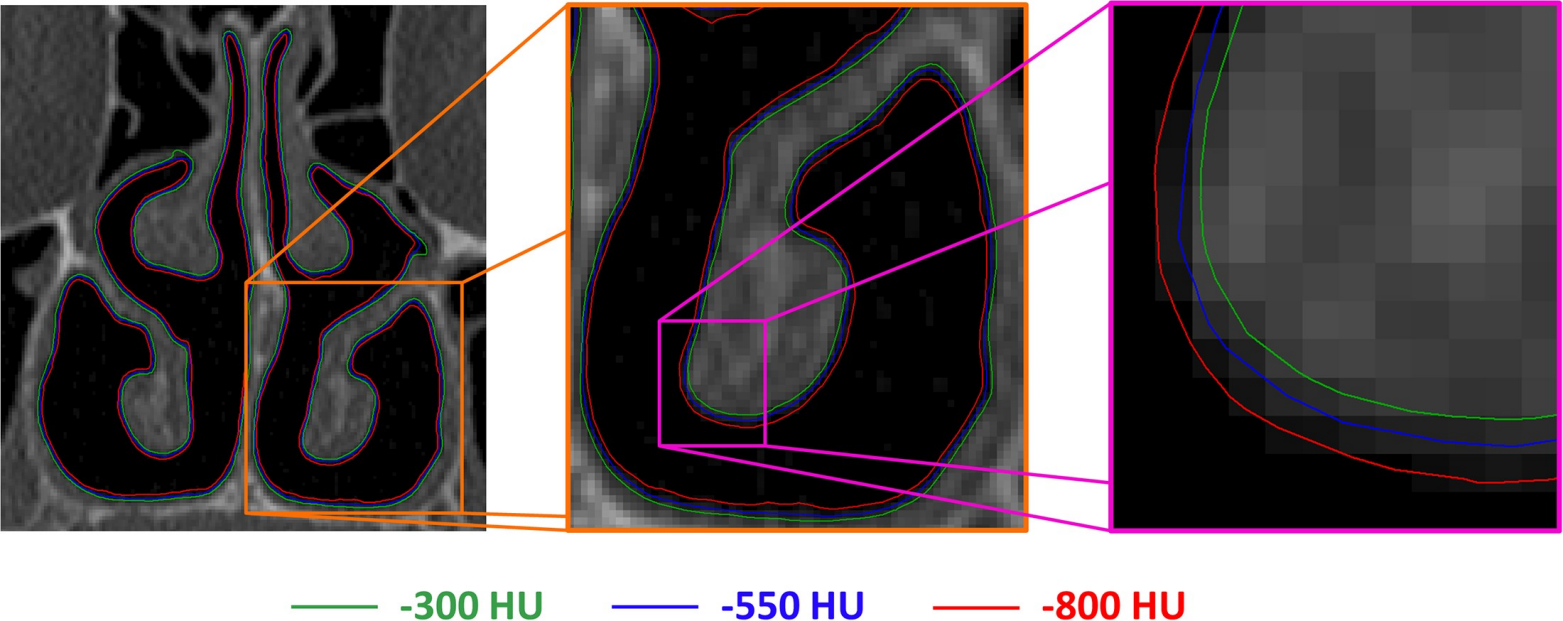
Outline of 3D reconstructions obtained with three different thresholds. Coronal CT scan of patient #1 showing the outlines of the 3D reconstructions created with segmentation thresholds -300 HU (green), -550 HU (blue), and -800 HU (red). The close-up view (right-side panel) reveals a nearly uniform distance of 1 to 2 pixels between the models created with segmentation thresholds -300 HU and -800 HU.
